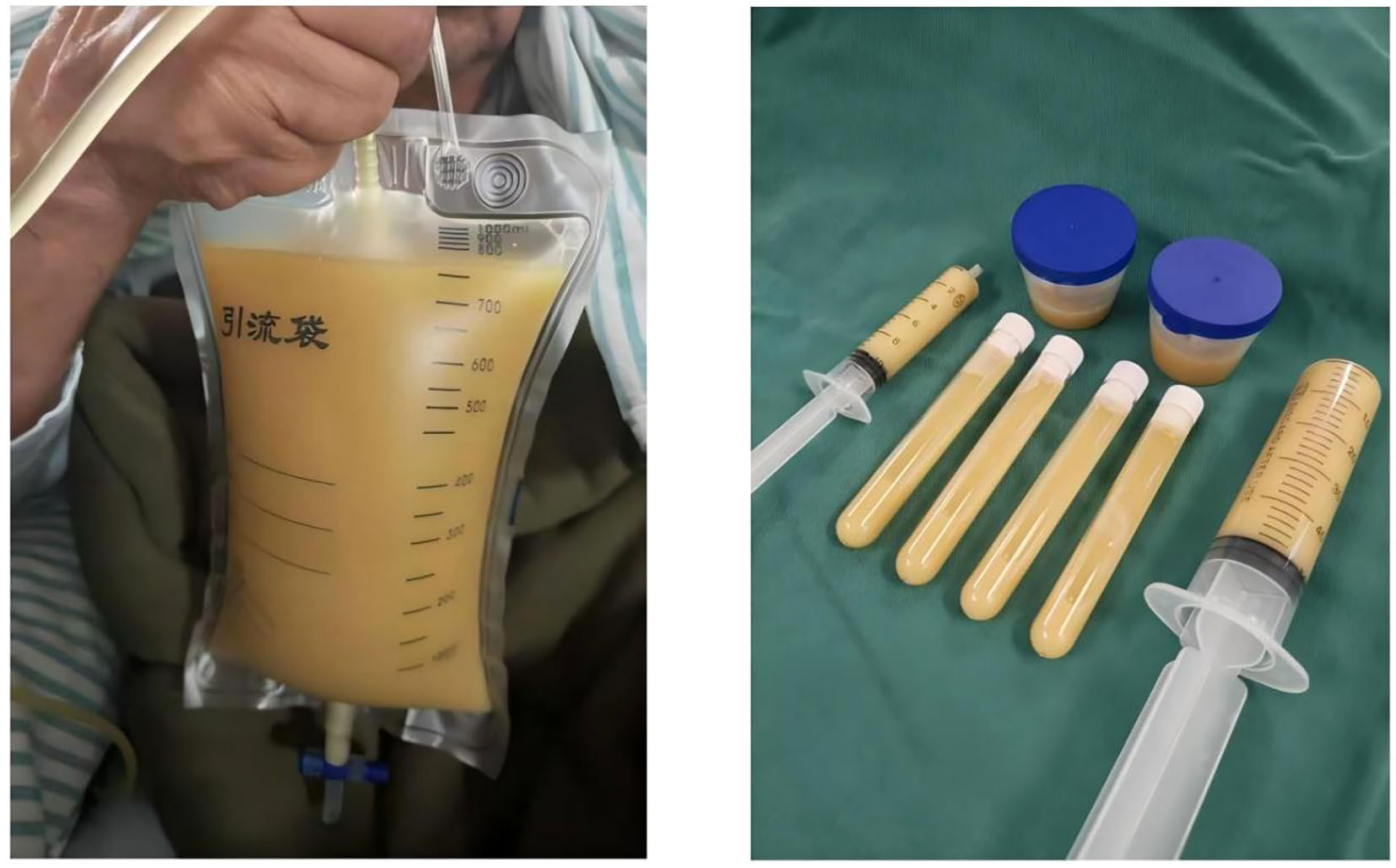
CT-guided percutaneous abscess drainage was performed, yielding a large volume of thick, purulent fluid.
